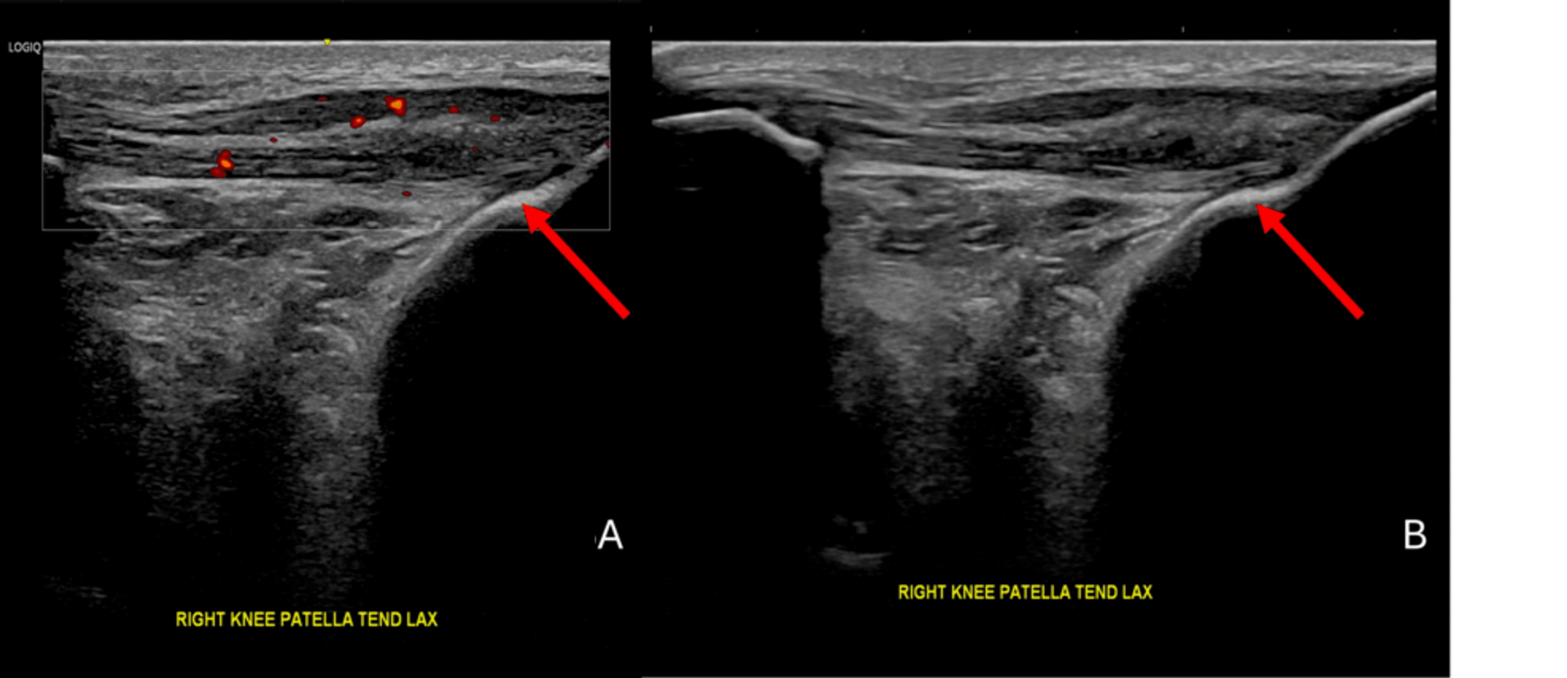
(A, B) Ultrasound images of the right knee showing healing of the patellar tendon with a hyperechogenic change in the tendon that was not compressible
